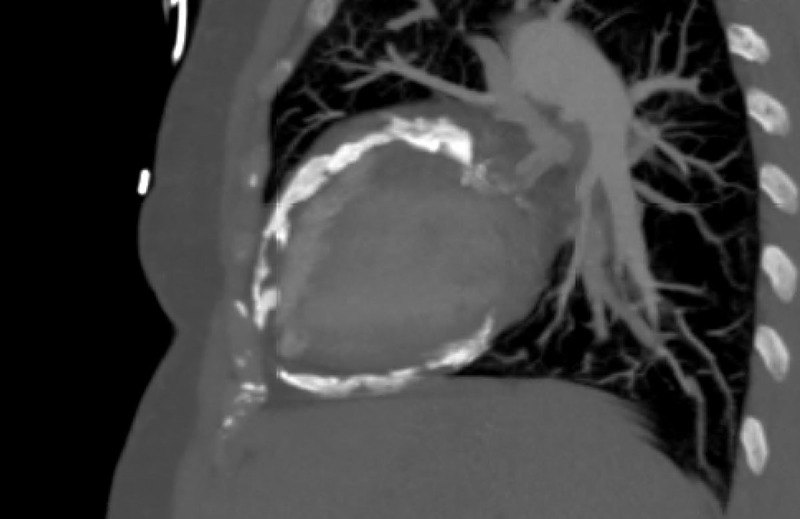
Sagittal view of severe pericardial calcifications on computed tomography.
